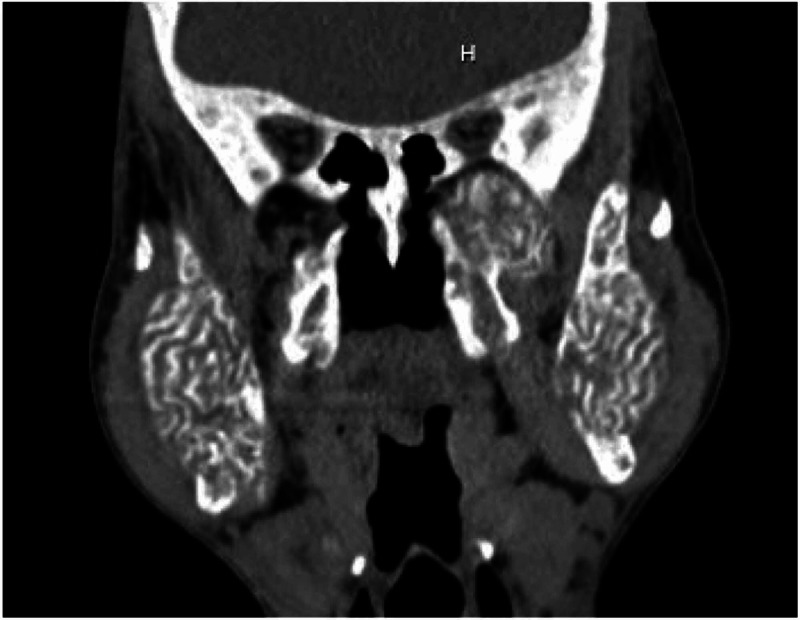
Coronal view - Non-contrast CT showing expanded mandible with prominent dense trabeculae.
